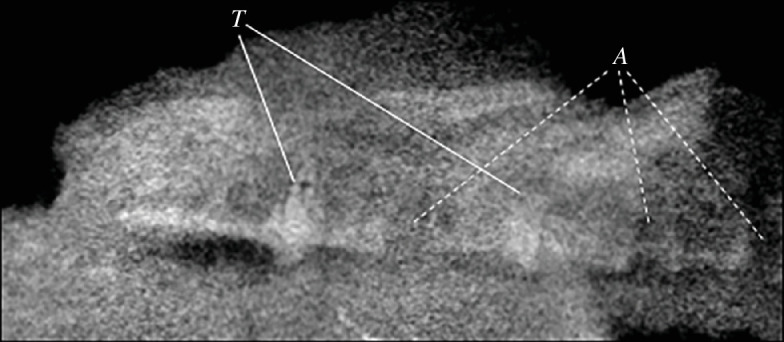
CT scan showing the position of two teeth (T*t*) scanned on the slab inside the maxilla, with three additional empty alveolar (A*a*) sockets.
